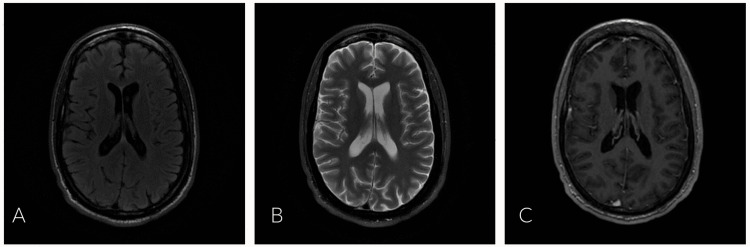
MRI brain: axial images with and without contrast A: MRI brain, axial view, T2 flair. B: MRI brain, axial view, T2 with fat suppression. C: MRI brain, axial view, three-dimensional fast spoiled gradient-echo with contrast. All images demonstrate no evidence of enhancing intra-axial or extra-axial neoplasm, demyelinating plaques, hemorrhage or acute infarction, hydrocephalus, or suspicious meningeal enhancement. Permission to reproduce obtained from the St. John's Riverside Hospital Department of Radiology and reviewed by co-author, Dr. Farid Abdo.
